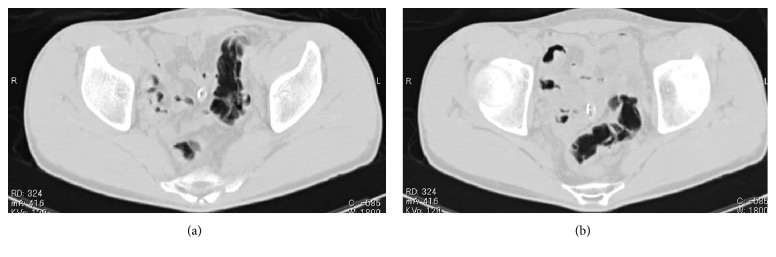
(a), (b) Repeat computed tomography image with an attenuation display. Reevaluation with an attenuation display revealing reticular strands from the distal transverse colon to the rectum probably corresponding to the foreign body.
